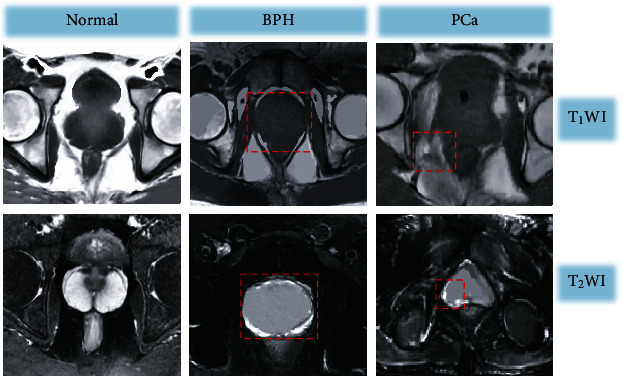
MRI plain scan images. The red circles marked the lesion regions.
